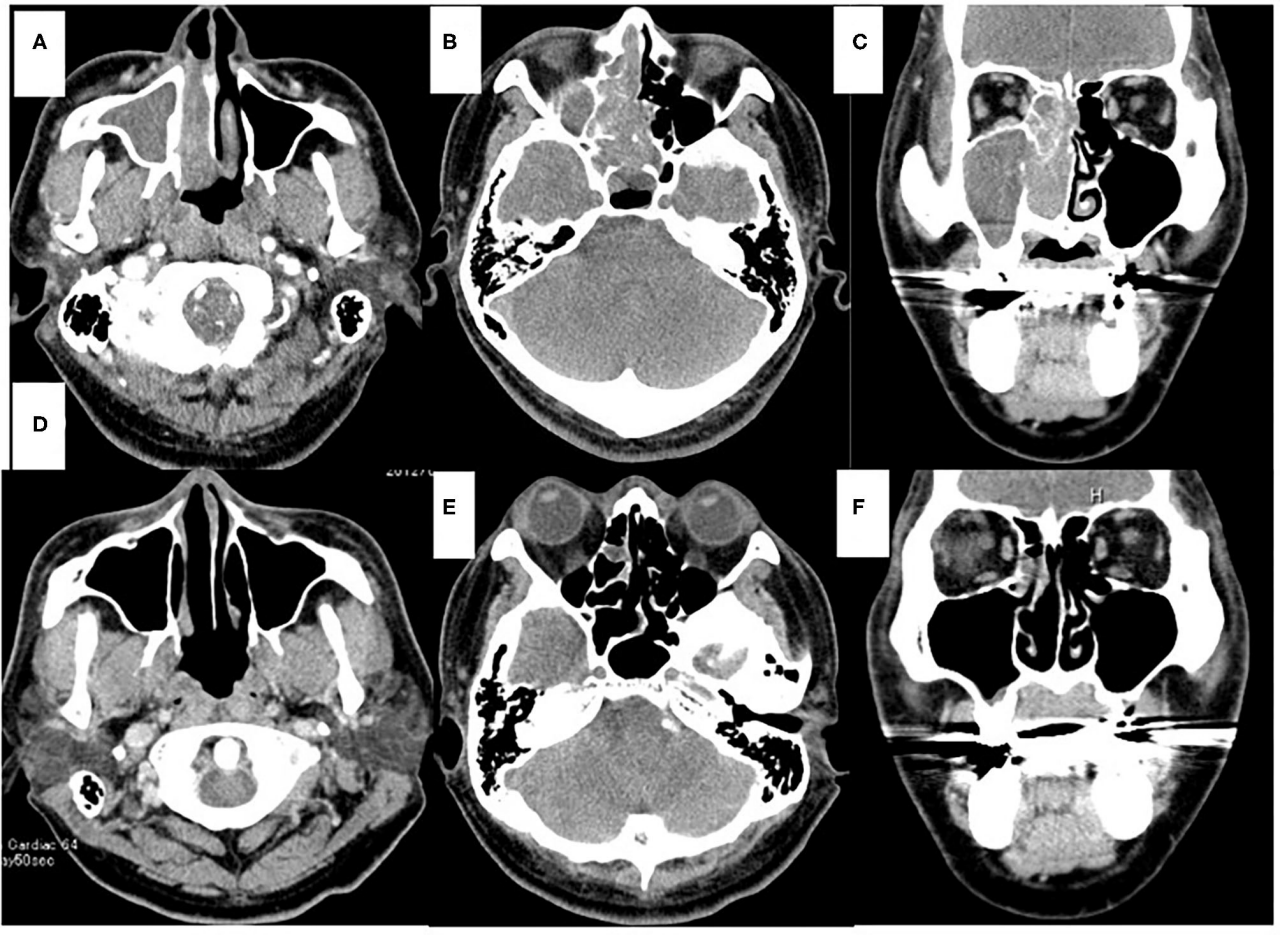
Computed tomography (CT) of the sinonasal region. Axial view (A,B,D,E). Coronal view (C,F). Enhanced CT scans obtained before chemoradiotherapy (A–C). On the right side, the nasal cavity and paranasal sinus were fully opacified. On the left side, the part of the sphenoid sinus were opacified. CT scans obtained after chemoradiotherapy (D–F). Opacification was only localized in the part of the right ethmoid sinus (E,F).
